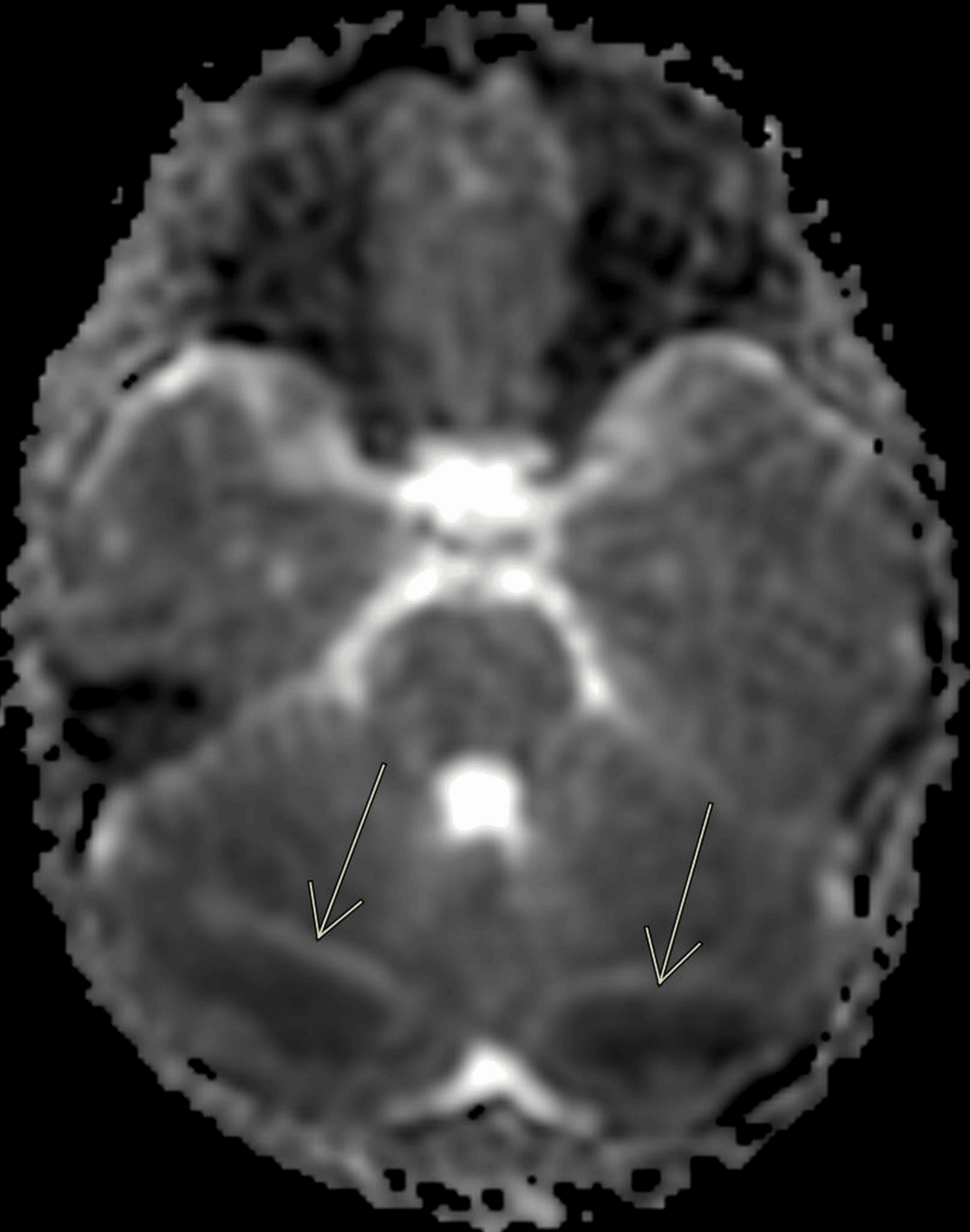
Brain MRI obtained on hospital day 3 Axial apparent diffusion coefficient (ADC) shows a bilateral hypointense signal in the cerebellar white matter, which confirms restricted diffusion.
